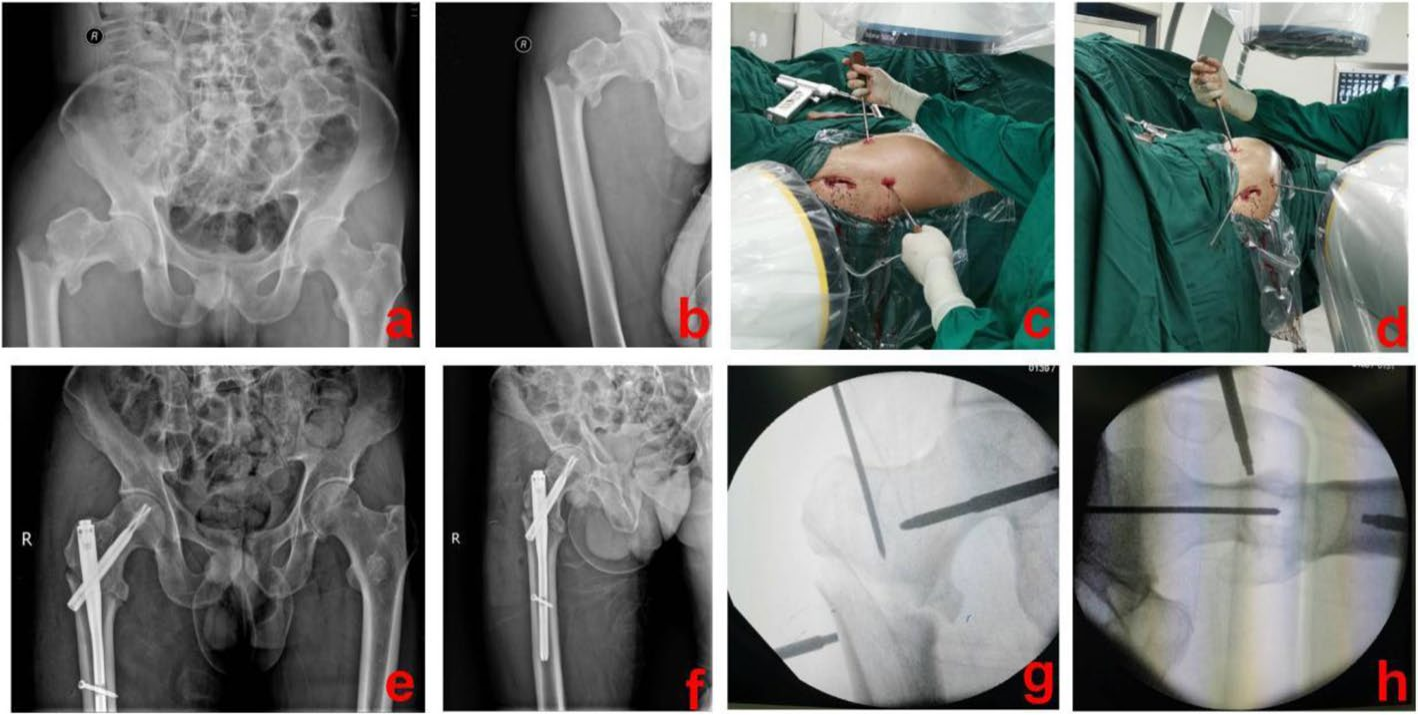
A 47-year-old male suffered from an extracapsular hip fracture and was treated using the PRDS technique. a and b Preoperative X-ray images of the injured hip; c and d During operation, PRDS technique was used for reduction; e and f Postoperative X-ray images showed that this fracture was well reduced and fixed; g and h Intra-operative X-ray images of the PRDS technique. PRDS stands for percutaneous reduction with double screwdrivers
